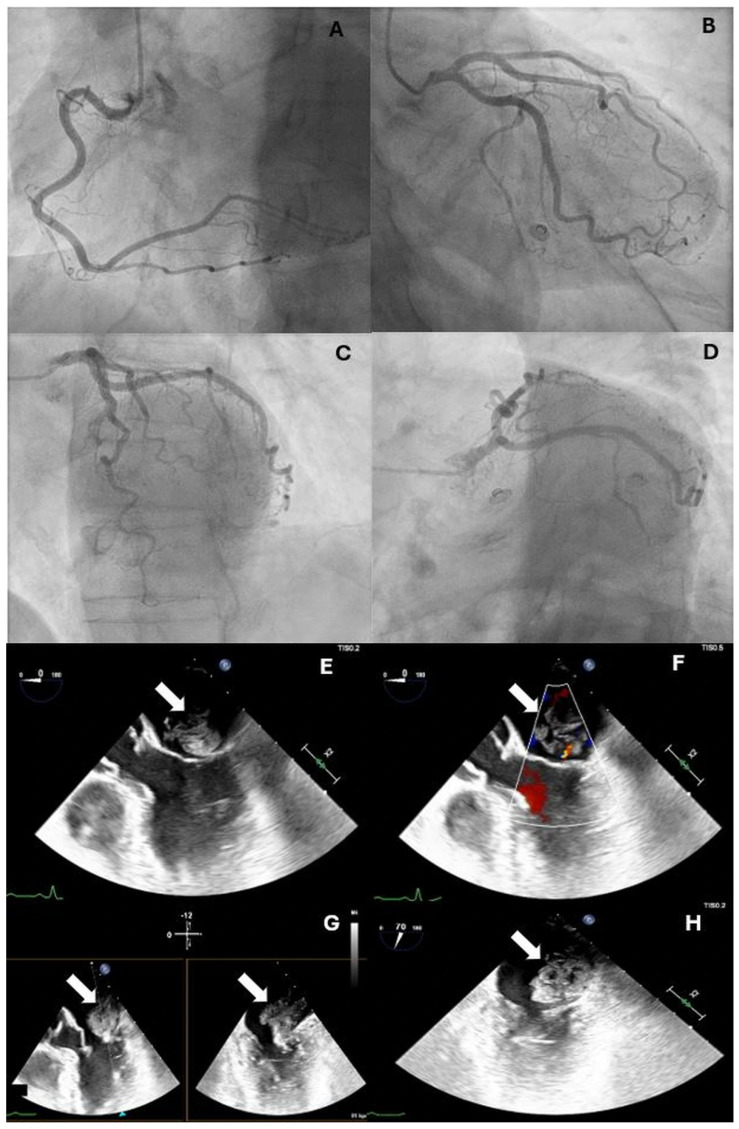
Coronary angiography and transesophageal echocardiography of left atrial myxoma. (A) Normal right coronary artery (RCA). (B–D) Normal left main stem (LMS), left anterior descending artery (LAD), and left circumflex branch (LCx). (E–H) Large mass with an irregular, heterogeneous appearance. The image reveals that it is attached by a stalk to the lateral wall of the left atrium. There is no evidence of vascularization.
